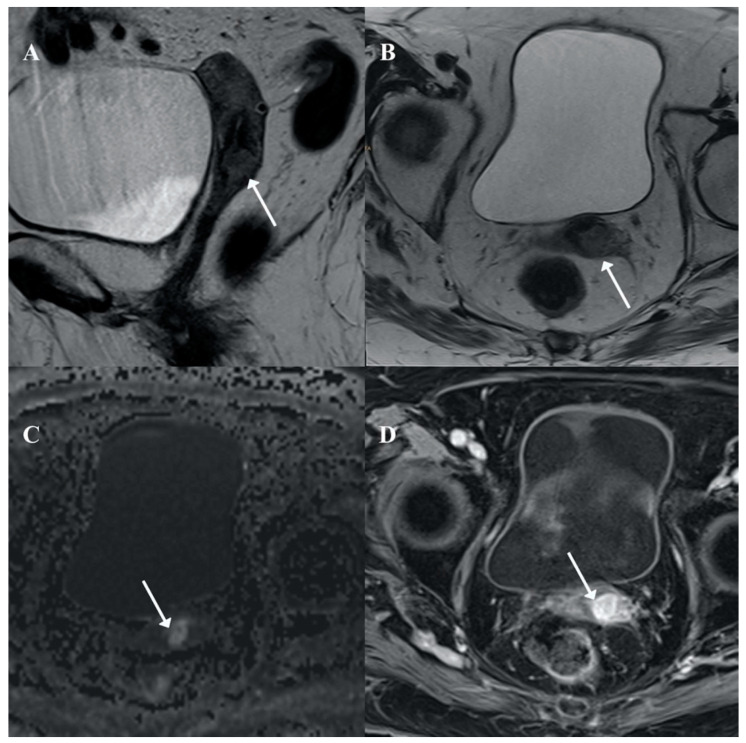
Pelvic magnetic resonance imaging (MRI). An infiltrative cervical mass (arrow) is seen in axial (A) and sagittal (B) T2 weighted MRI with stromal infiltration as a disruption of the cervical stroma hypointensity and focal infiltration of superior vaginal third (arrow in A). The lesion showed restricted diffusion in high b value (C: DWI b 800) and contrast enhancement (D: Post-contrast axial T1w fat saturated MRI).
